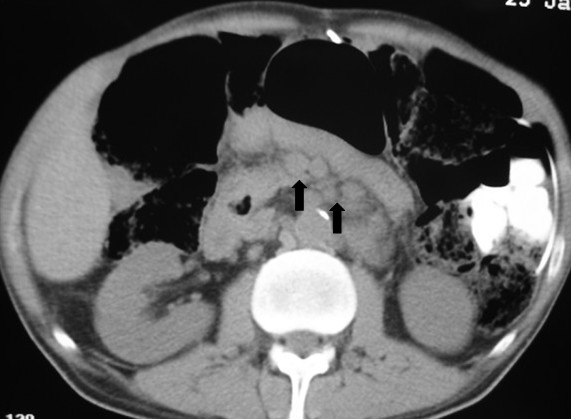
An abdominal computed tomography scan showing para-aortic lymphadenopathy.
